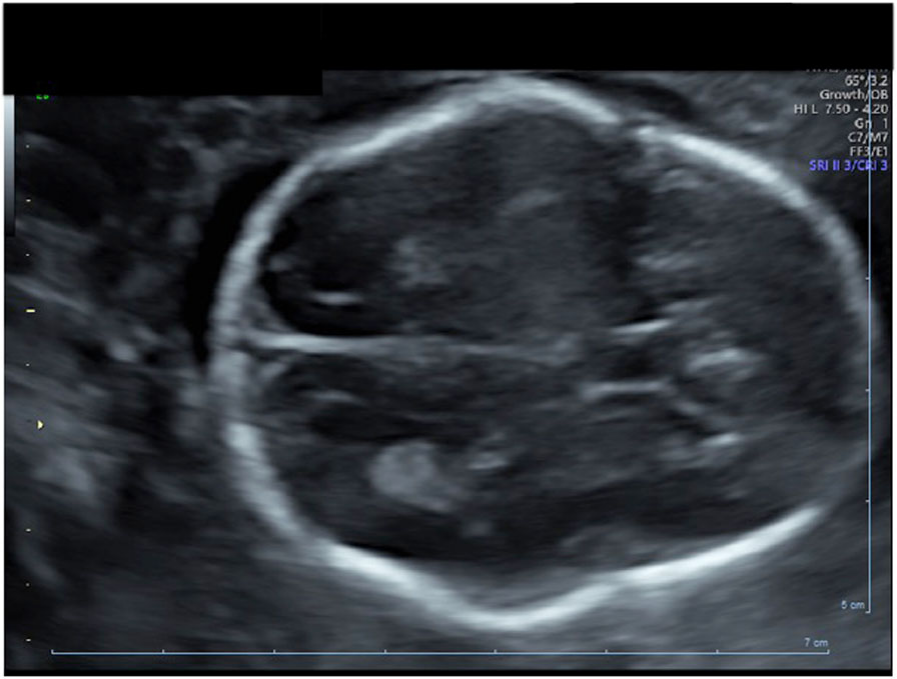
Ultrasound images demonstrate abnormal skull shape with open cranial sutures at 20 weeks gestation
